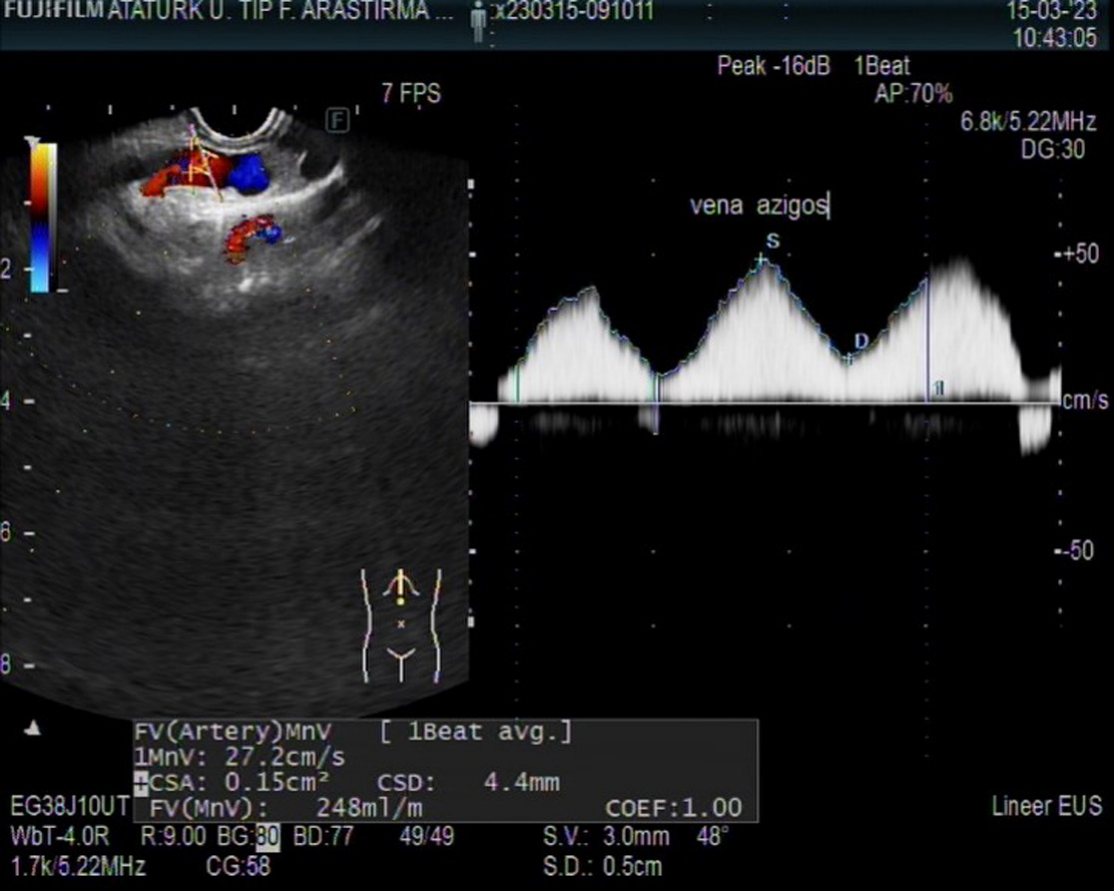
Vena azygos velocity and Doppler spectrum.
